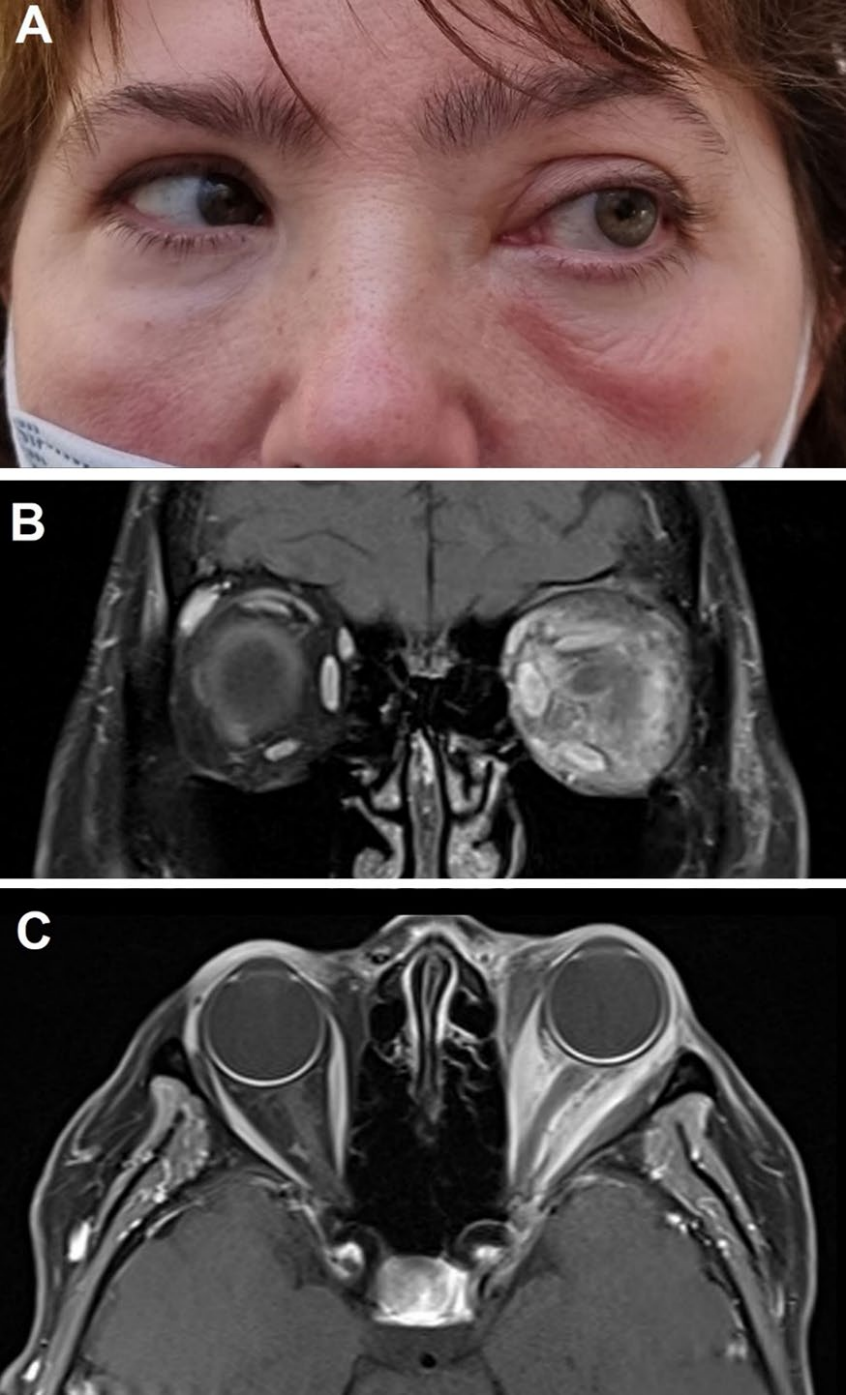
Clinical photographs and MRI orbits of Case 3. A demonstrates the surrounding periorbital oedema and erythema, with proptosis and restricted left eye abduction. B (coronal) and C (axial) demonstrate T1-weighted, fat-suppressed, contrast-enhanced images showing proptosis and diffuse extra- and intra-conal fat enhancement extending close to the orbital apex and superior orbital fissure, with enlargement and peripheral enhancement of all the EOM
